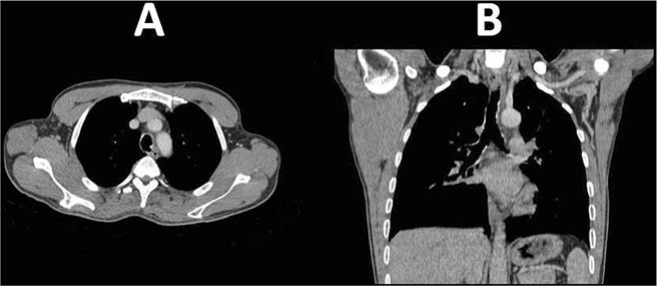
Chest computed tomography scan: Axial sections (A) and coronal reconstruction (B) showing thickening and calcified lesions of the trachea.
